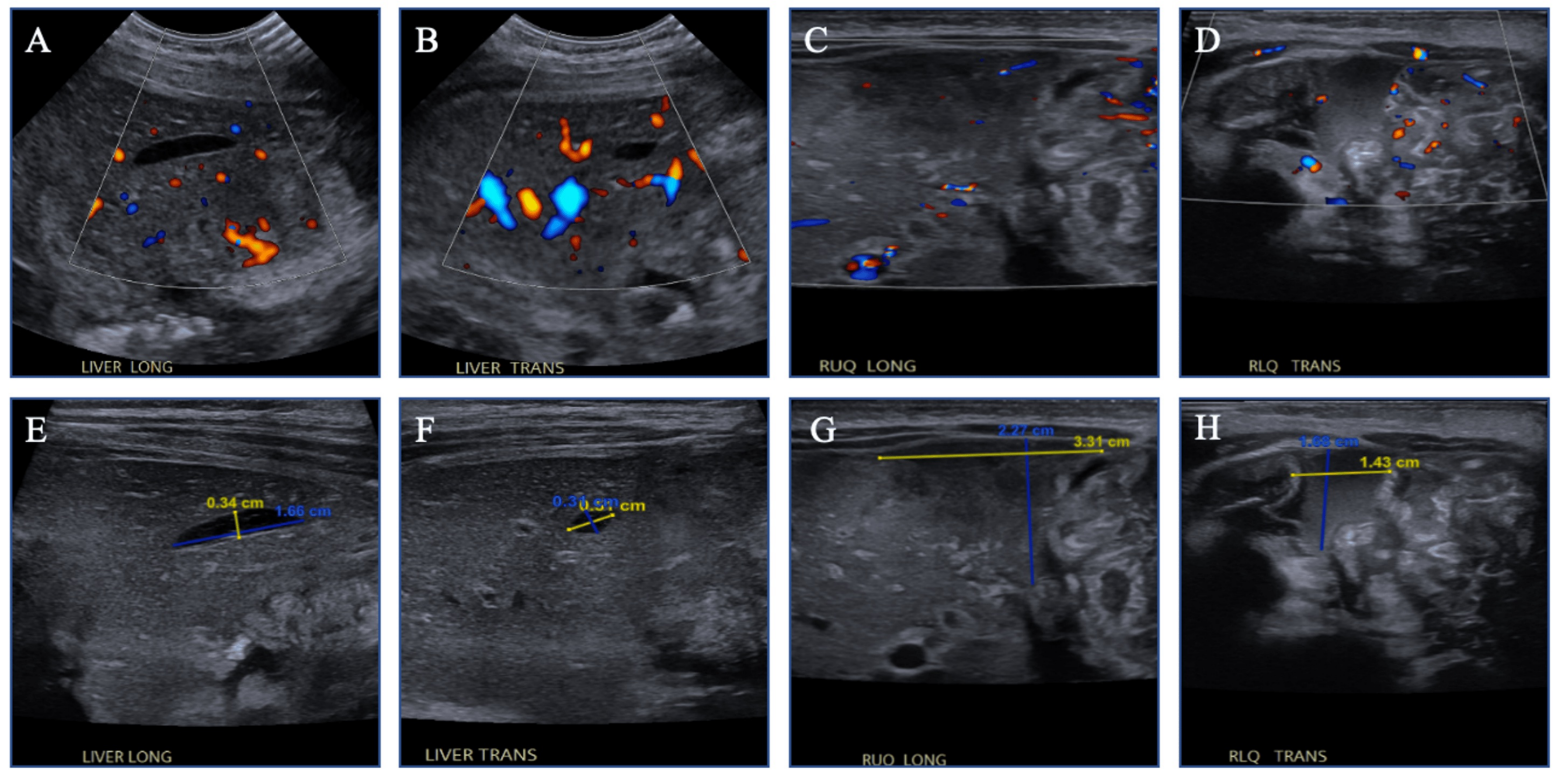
Abdominal ultrasound demonstrated subcapsular and intrahepatic abscesses with mass effect on the left lobe of the liver as well as on adjacent bowel loops and diffuse bowel wall thickening. (A) Liver long axis; (B) liver transverse axis; (C) right upper quadrant long axis; (D) right lower quadrant transverse axis views with Doppler showing vasculature; (E) liver long axis; (F) liver transverse axis view showing intrahepatic abscesses; (G) right upper quadrant long axis view demonstrating mass effect on left lobe of liver and adjacent bowel loops and diffuse bowel wall thickening; (H) right lower quadrant transverse axis view showing diffuse bowel wall thickening and complex free fluid in the peritoneal cavity.
